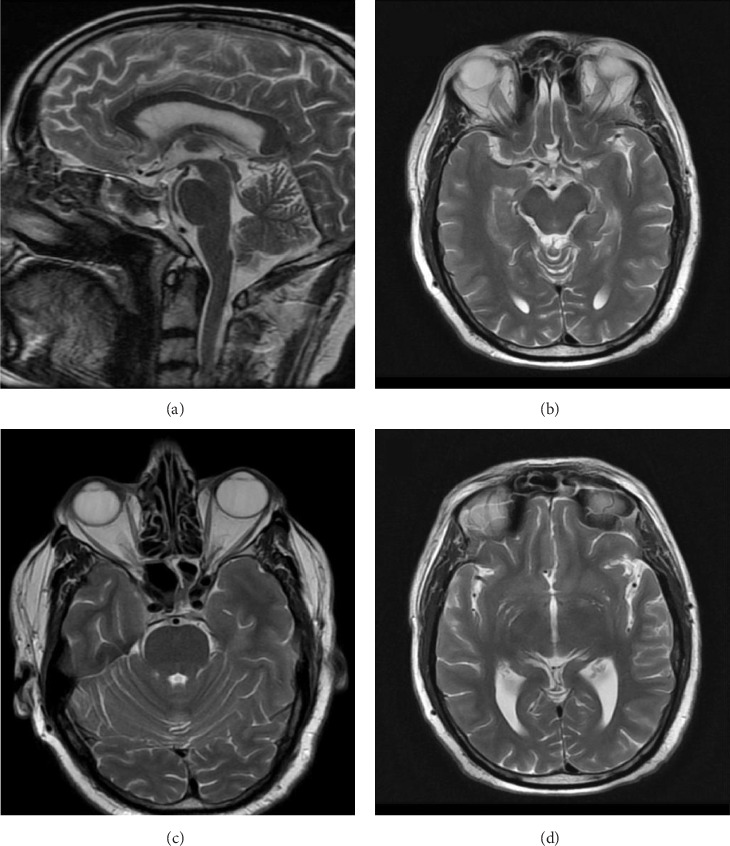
MRI brain scans of the patient (a) sagittal T2-weighted image showing the midbrain and brainstem with preserved pontine and cerebellar structures. (b) Axial T2-weighted image at the basal ganglia level, with no significant putaminal atrophy or hypointensity. (c) Axial T2-weighted image at the midbrain level, where substantia nigra changes may be seen in PD on advanced sequences. (d) Axial T2-weighted image showing mild cortical atrophy, which can be seen in advanced PD.
